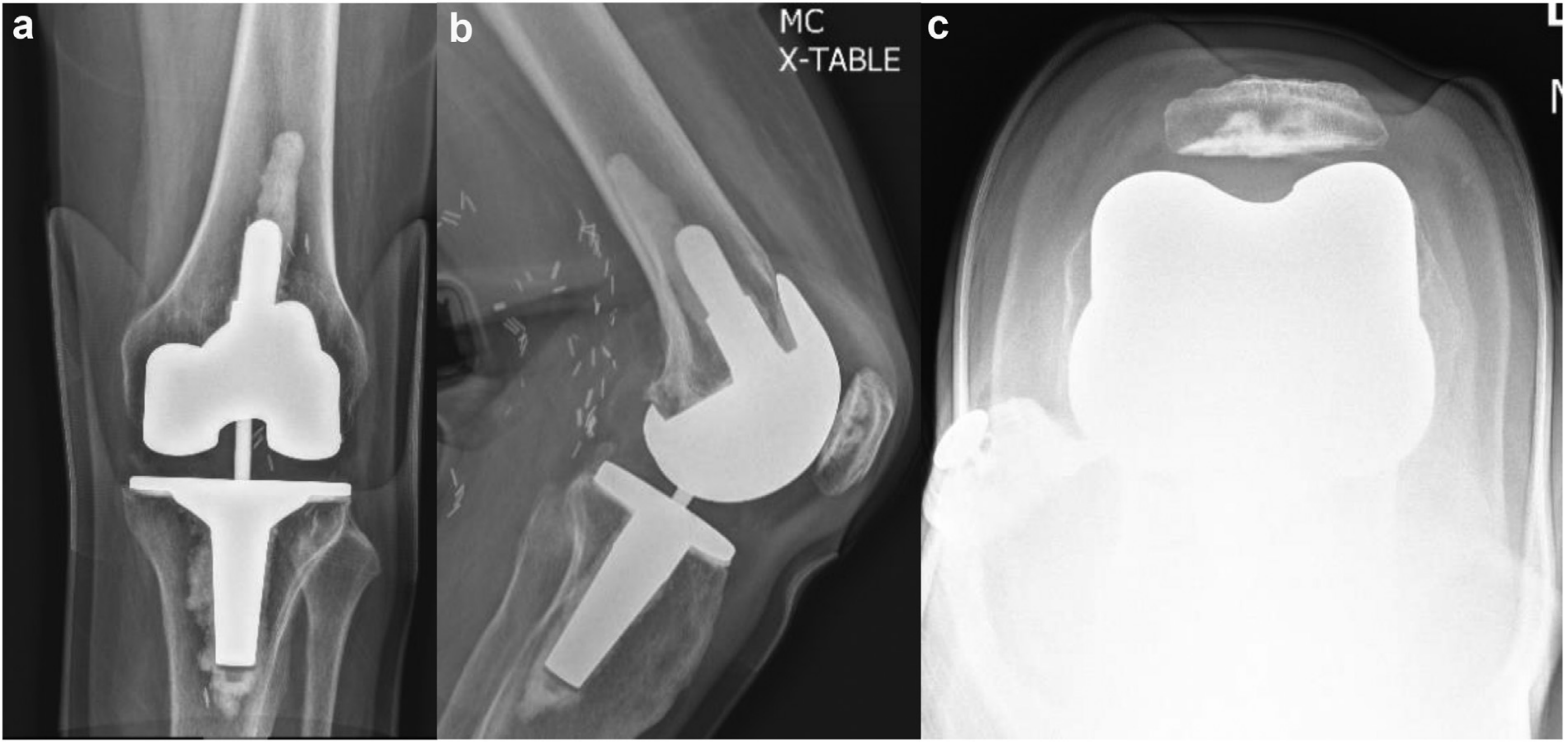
Postoperative radiographic imaging of the right knee: (a) anteroposterior, (b) lateral, (c) sunrise view.
